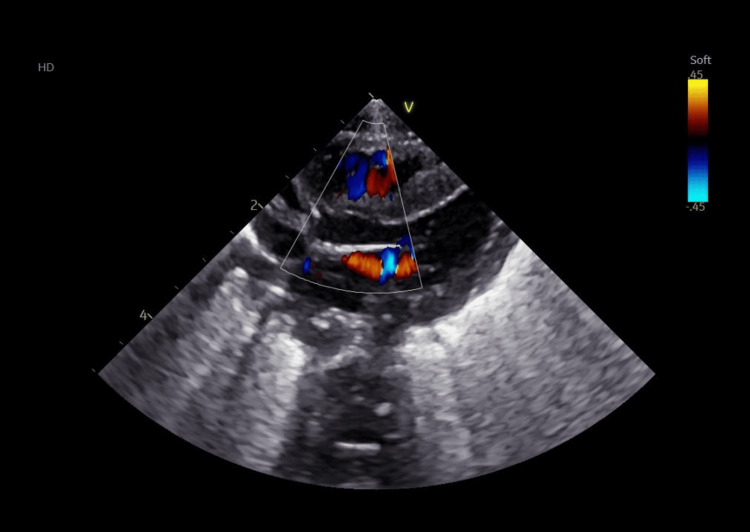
Echocardiography: Color Doppler of the aortic outflow tract showing no tract obstruction
